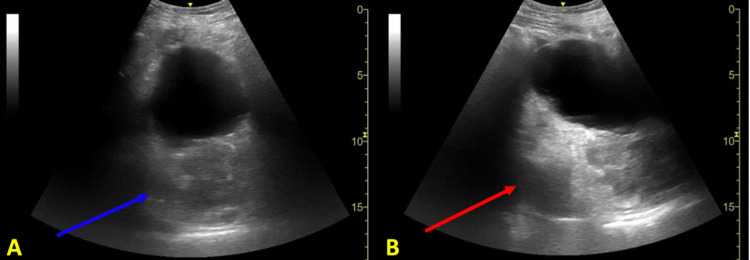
Sonographic image of the suprapubic region Bedside POCUS image utilizing a low-frequency curvilinear probe at the suprapubic region with transverse (A) and sagittal (B) views showing the bladder with posterior acoustic enhancement. There is a poorly defined, heterogenous mass lesion deep to the bladder in the rectosigmoid region measuring approximately 7 cm (blue arrow). There is evidence of colonic dilation proximal to the mass lesion (red arrow).
